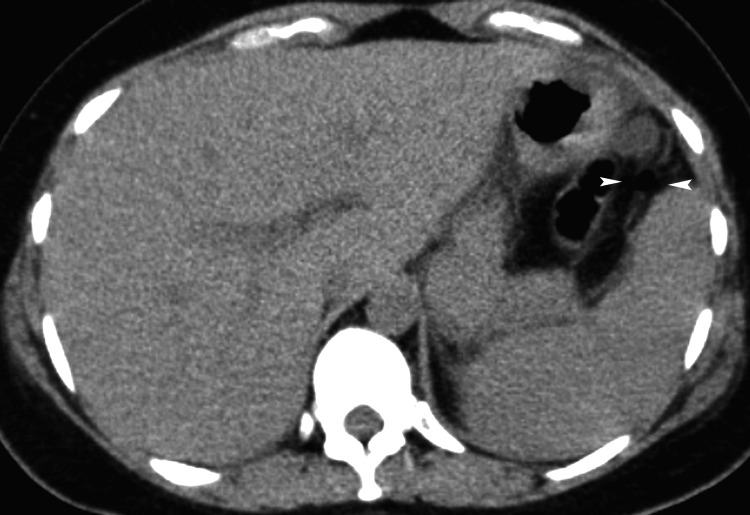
Axial computed tomography (CT) image CT image shows free air (arrowheads) in the peritoneum.
